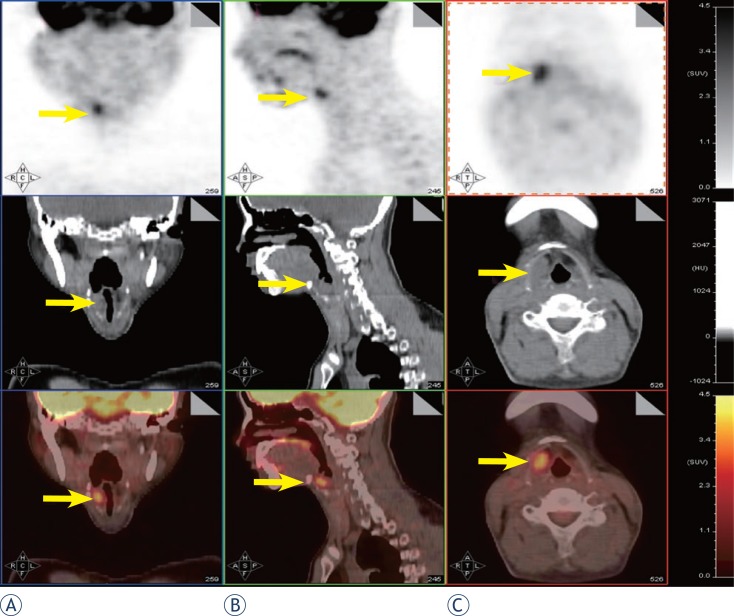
PET/CT showed high FDG uptake in the region of the right hypopharynx and laryngeal inlet (SUV_max_ = 4.1) and no distant metastasis.
